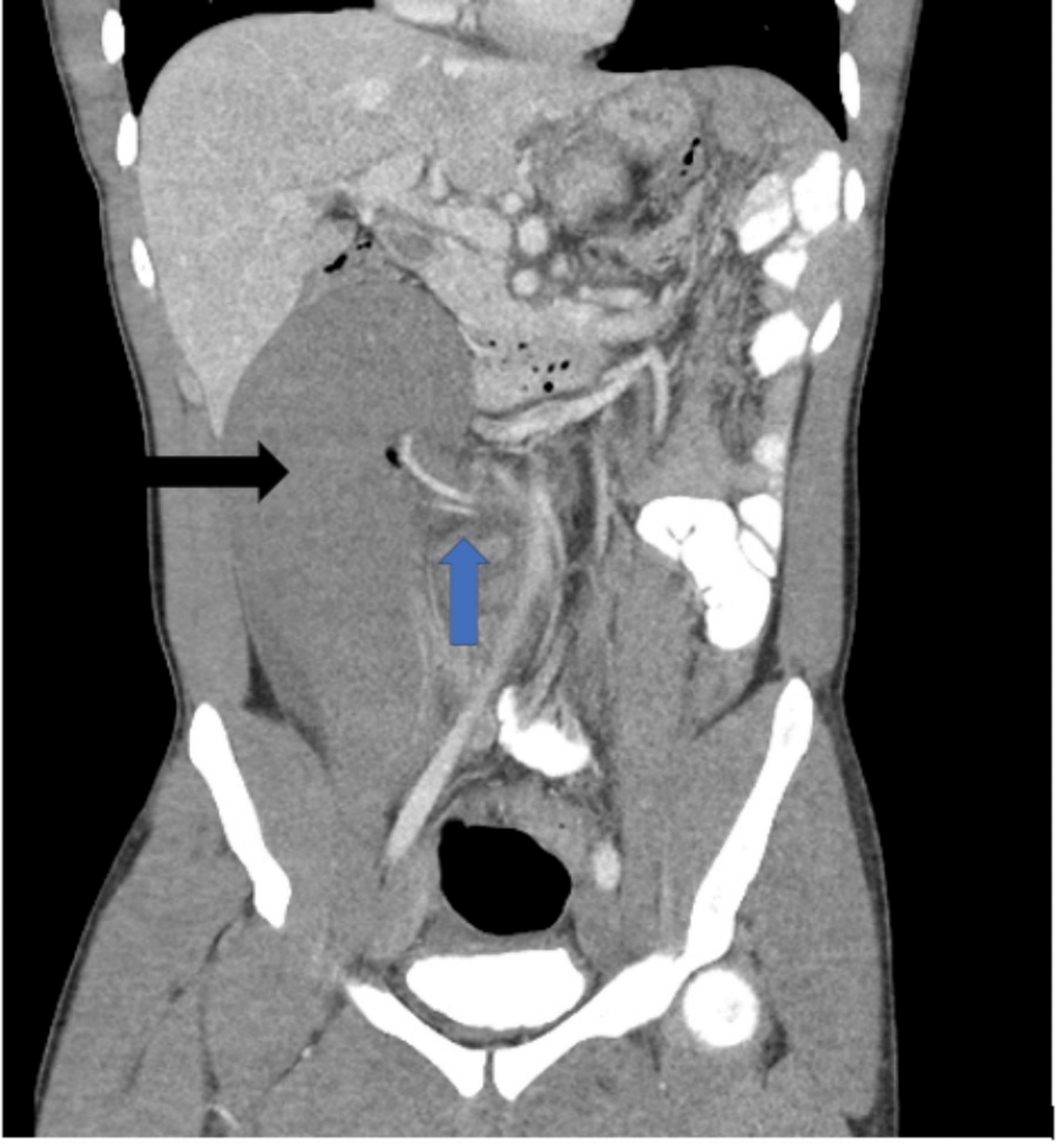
CT abdomen and pelvis, coronal view, slice 34. Black arrow representing wandering spleen. Blue arrow demonstrating splenic pedicle.
